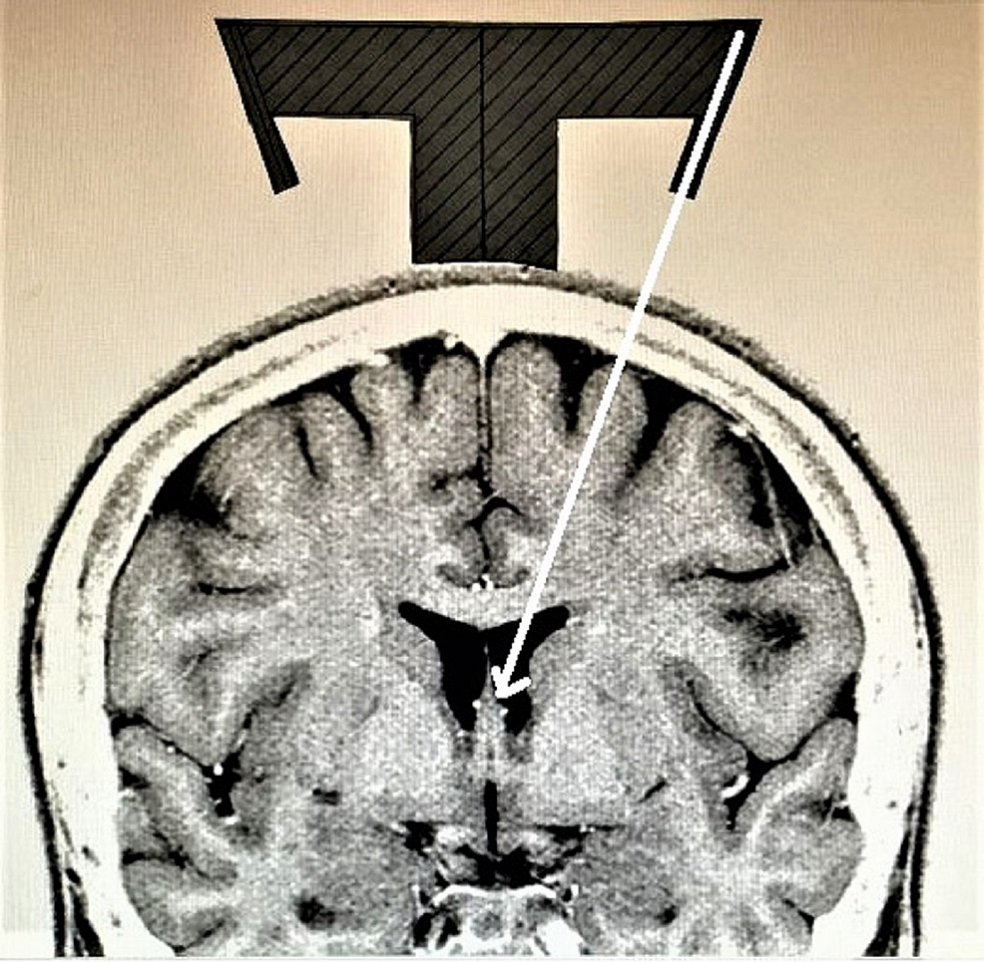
Shows the template of the guide on top of the head on a coronal MR image with the probe holder aimed towards the foramen of Monro
